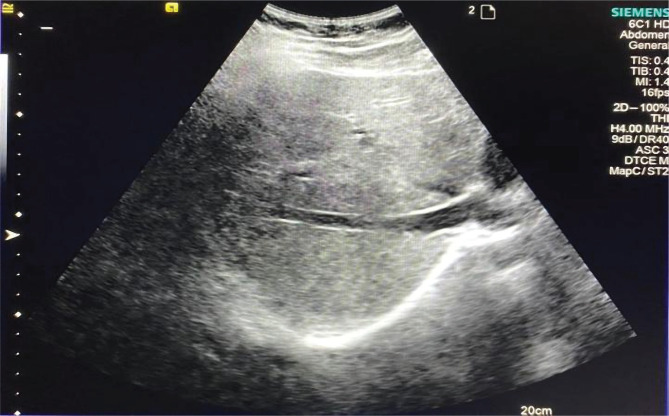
Sonogram of the liver in patient M., 56, with DTG. Heterogeneity of the parenchyma echostructure.
